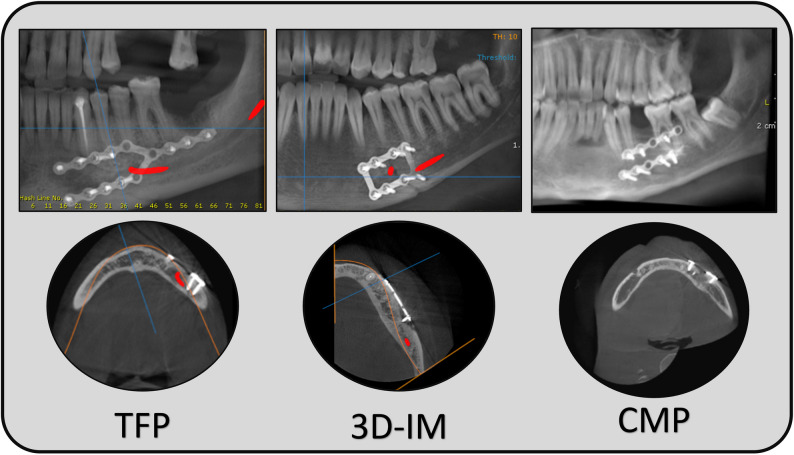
Postoperative radiographic assessment for the three miniplates configurations. Bone density analysis was conducted by the application of six 3_ × _3 regions of interest along the fracture line, where the mean value for each record was calculated
